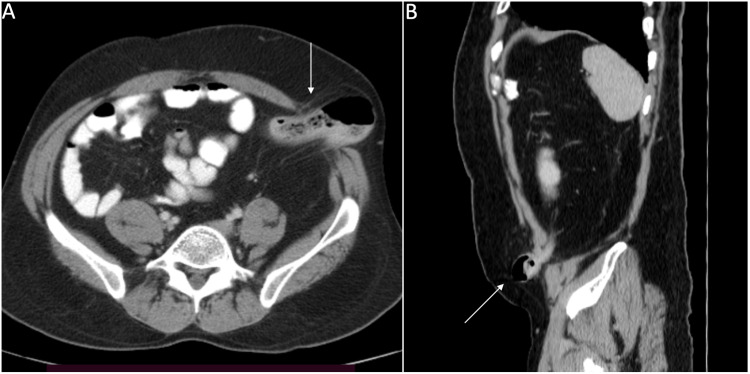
Contrast-enhanced CT images. A: Axial image showing the herniating sigmoid colon and the defect in the abdominal wall. B: Sagittal image showing the protruding sigmoid colon.
